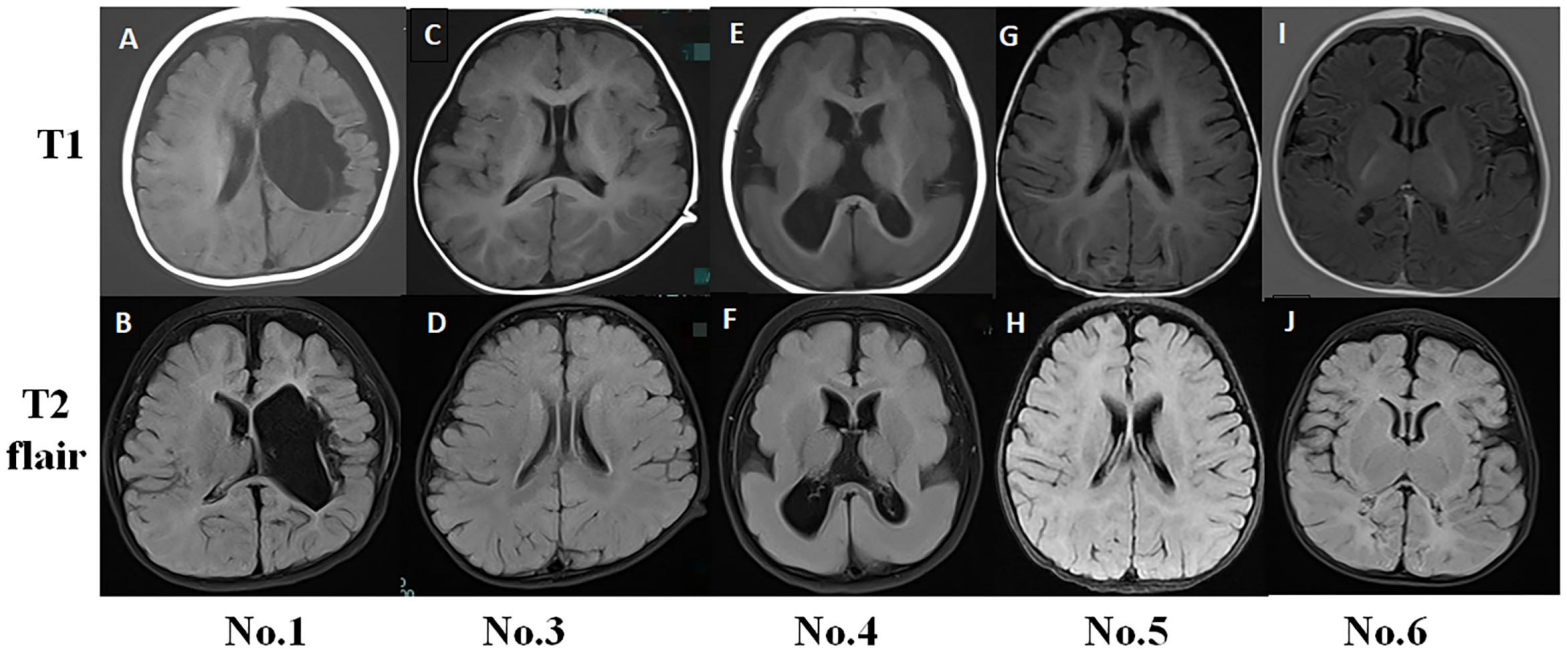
Typical magnetic resonance imaging (MRI) findings in infantile spasm (IS) patients. (A,B) No. 1 MRI (8-month-old) showed left hemisphere softening, left ventricle deformity, and cerebral peduncle atrophy. (C,D) No. 3 MRI (15-month-old) had normal findings. (E,F) No. 4 MRI (11-month-old) showed pachygyria. (G,H) No. 5 MRI (6-month-old) had normal findings. (I,J) No. 6 MRI (4-month-old) exhibited abnormal signals in the bilateral occipital and parietal lobes.
